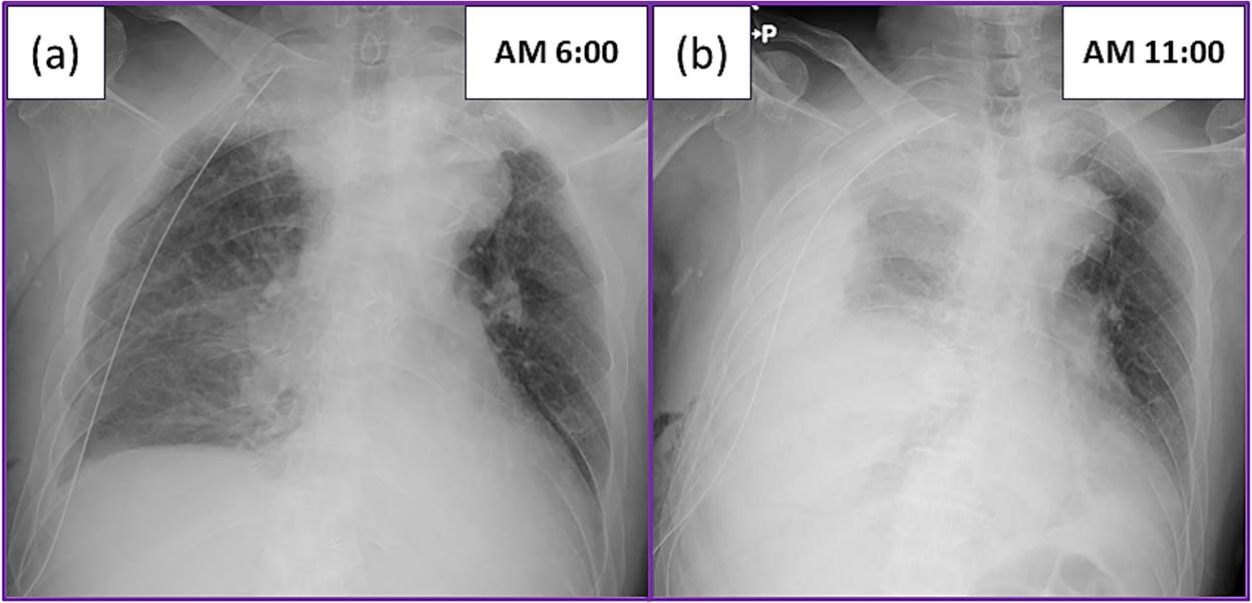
Postoperative chest X-ray: (a) Chest X-ray on the morning after surgery showed the expected postoperative changes. (b) The patient suddenly complained of dyspnea, and a large amount of bloody effusion drained from the chest tube after mobilization. The chest X-ray showed a large amount of effusion.
